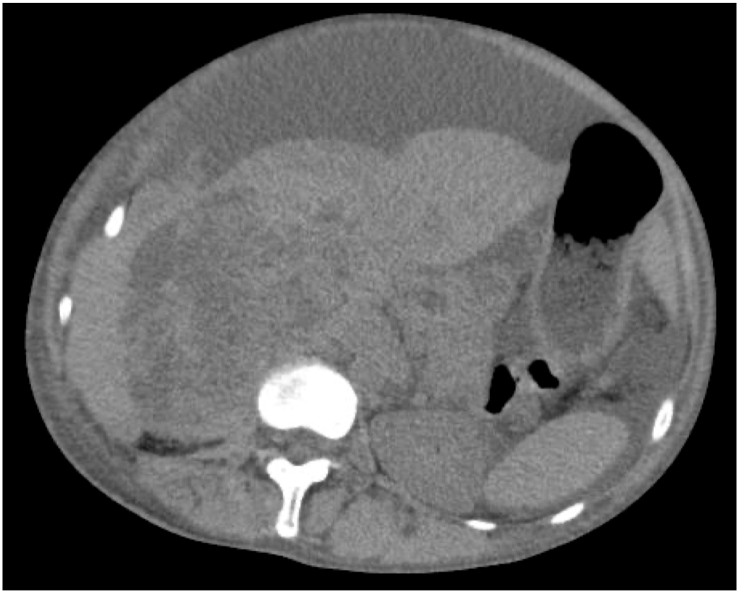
Abdominal CT image on the first visit to our hospital showing a large right hepatic mass of irregular density with indistinct borders. Abundant ascites was also detected.
